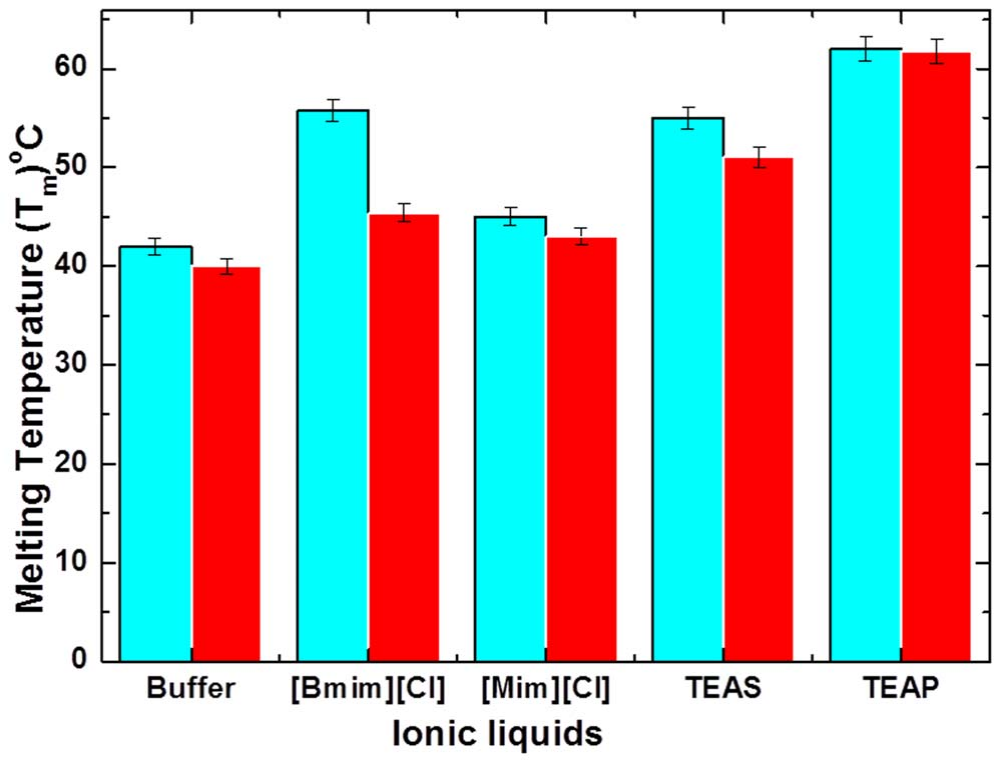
The variation in melting temperature (T_m_) values of CT in buffer and ILs (a) without APPJ treatment (cyan colour) and (b) with APPJ treatment (red colour). The data points are average values of atleast three determinations, the error bars indicating ± mean deviation.
